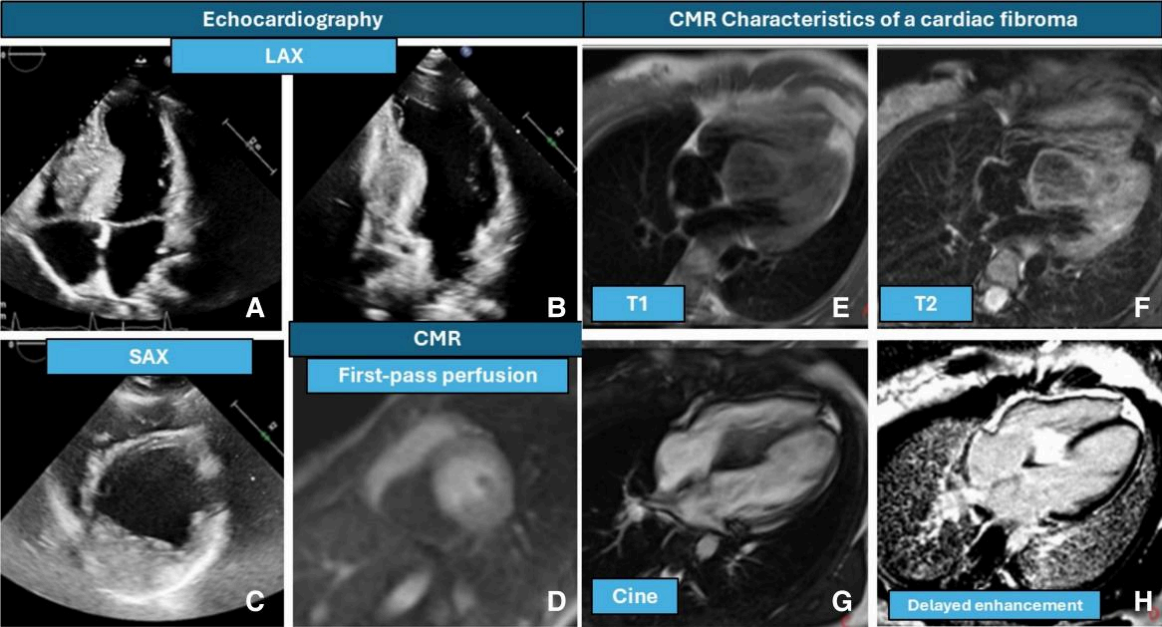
(*A*) Transthoracic echocardiography apical 4C with image compatible with fibroelastoma in the lower septal region. (*B*) Transthoracic echocardiography apical 2C with fibroelastoma in basal inferior segment. (*C*) Short-axis echocardiography, image compatible with fibroelastoma basal inferoseptal. (*D*) First-pass perfusion sequence, basal short-axis plane without initial uptake by the mass. (*E*) T1-weighted spin-echo sequences, hypo-intense/isointense mass with respect to myocardium. (*F*) T2-weighted black blood sequences with hypo-intense/isointense mass compared with healthy myocardium. (*G*) Cine sequences, with image of mass in the lower septum. (*H*) Delayed enhancement study, the mass is uniformly hyper-intense.
